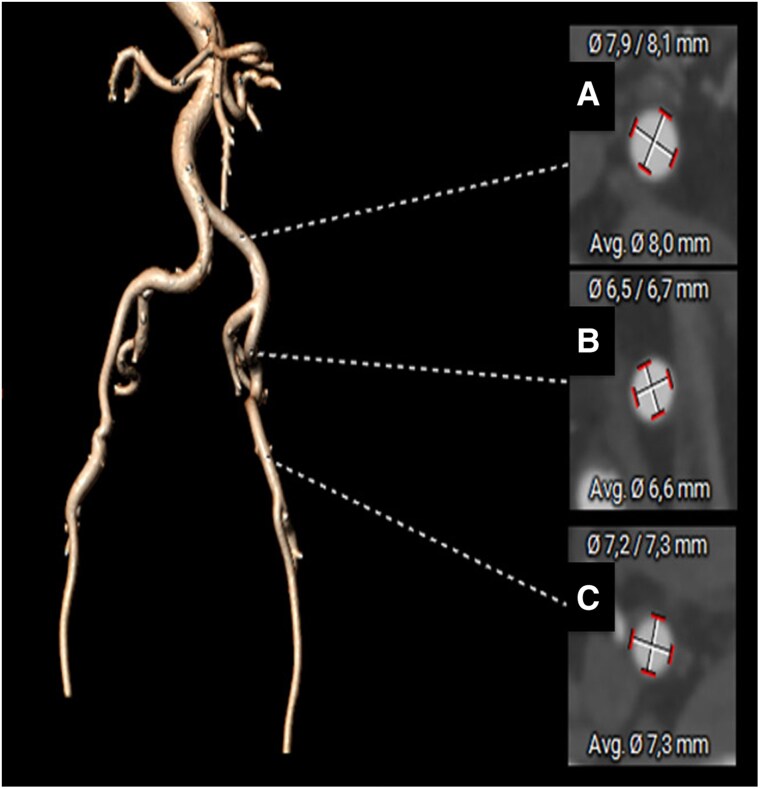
At computed tomography, iliofemoral arteries reconstruction. (*A*) Dimensions at the left common iliac artery level; (*B*) dimensions at the left external iliac artery level; (*C*) dimensions at the common left femoral artery level.
